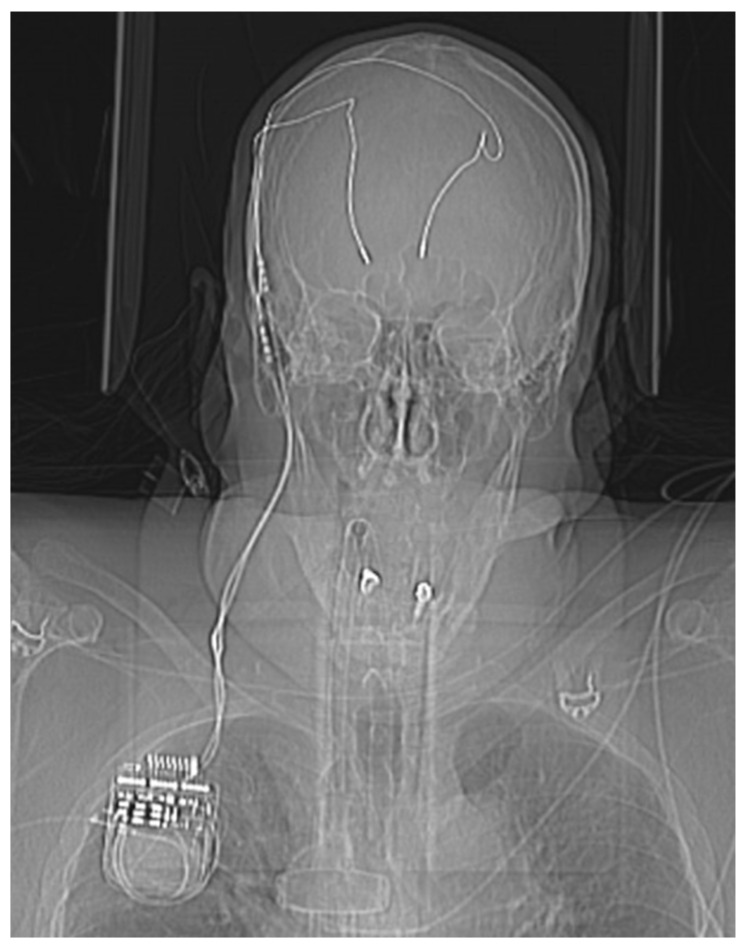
Initial scout image of the head CT scan, essentially mimicking an AP cranial X-ray. Note the implanted stimulations leads for deep brain stimulation, which are connected to an infra-clavicular neurostimulator. The DBS stimulation leads are displaced to the right side.
